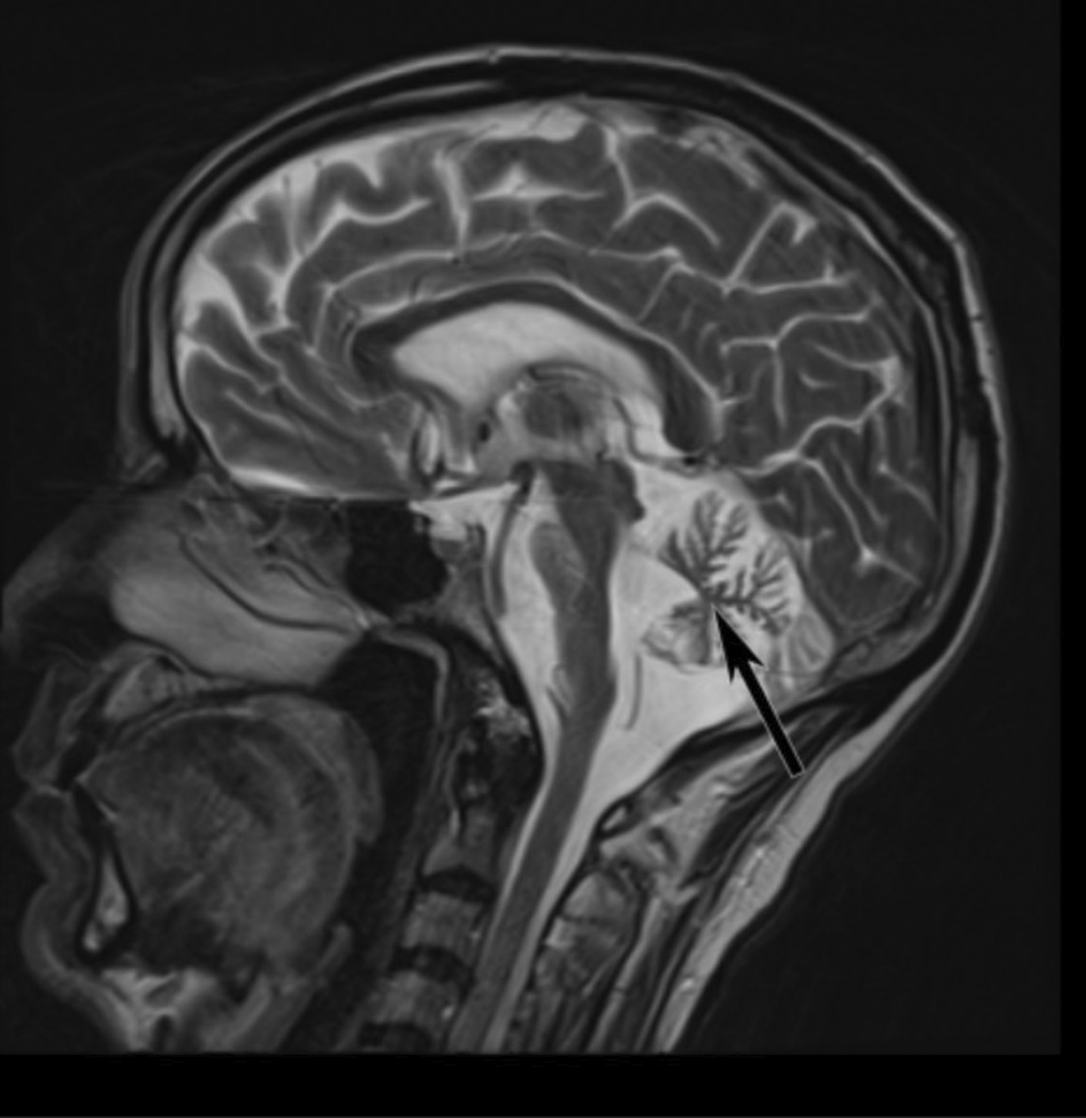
Cerebellar degeneration on T2‐weighted MRI (black arrow).
